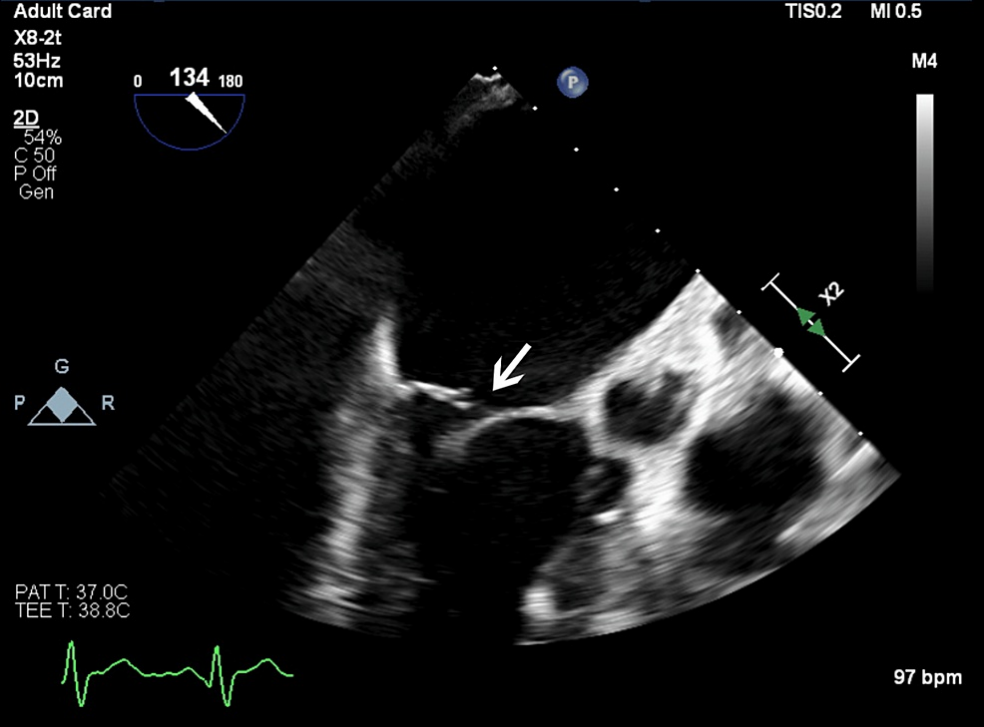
Transesophageal echocardiography image highlighting rupture of the posterior leaflet of the mitral valve causing mitral regurgitation.
